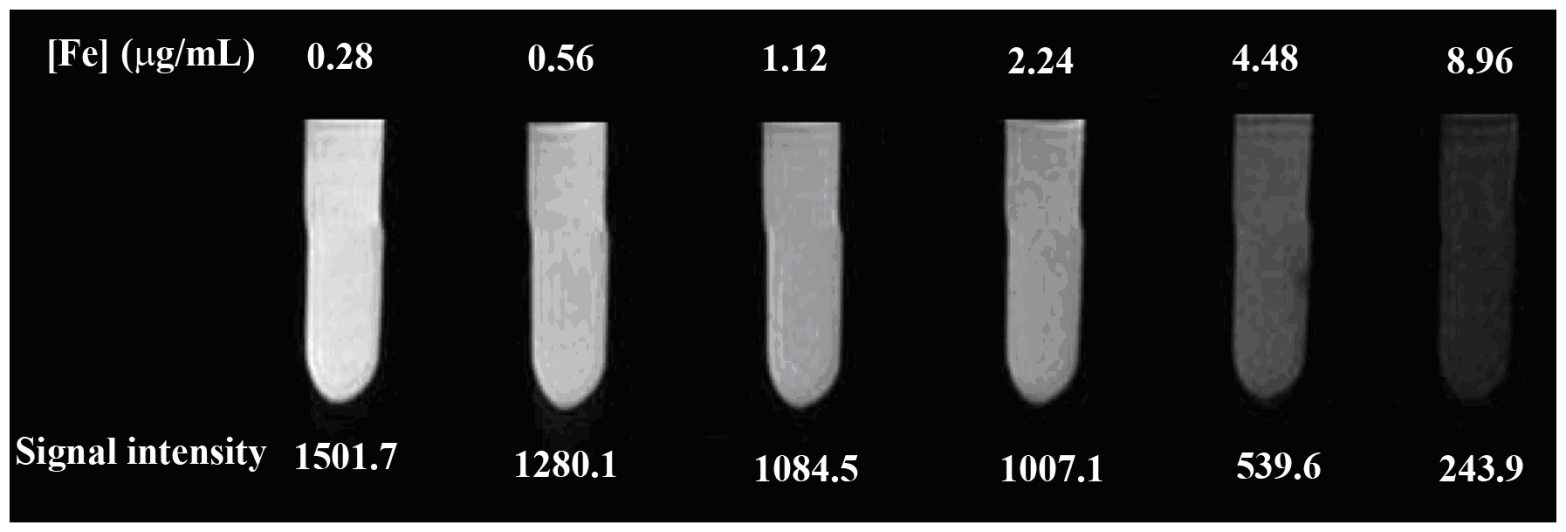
The T_2_-weighted MR images and signal intensity of the HA-Fe_3_O_4_ NPs at different Fe concentrations.
